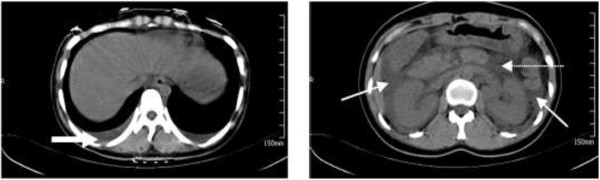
Thoraco–abdominal CT images of a 22 year-old woman with HFRS complicated with SAP (case 2). Pleural effusions (thick white arrow), peripancreatic fluid collection (thin white arrow), and peripancreatic fat stranding (dotted-line white arrow) are shown.
